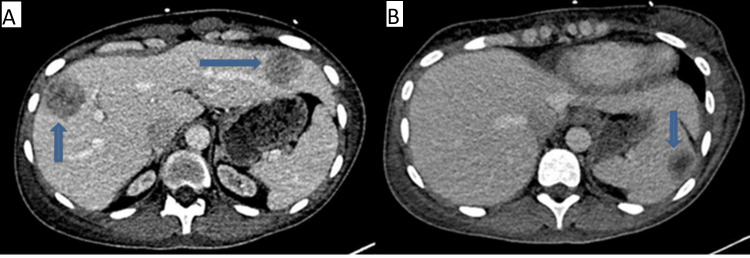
A. CT Abdomen revealed multiple solid hypoenhancing hepatic lesions with the largest measuring 3.7x3.5 cm in the right hepatic lobe and B. hypoenhancing splenic lesion measuring 2.7x2.1 cm. (blue arrows)
